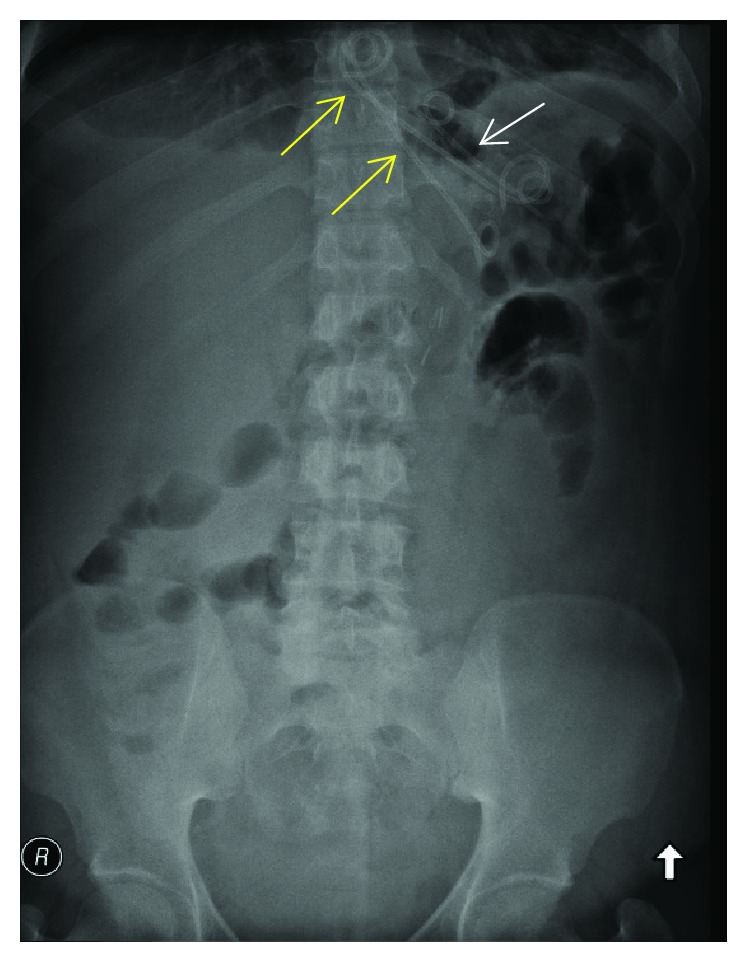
X-ray abdomen showing the displacement of one double-pigtail drain into the collection (white arrow). A satisfying double-pigtail drain position across the leak orifice (yellow arrows).
